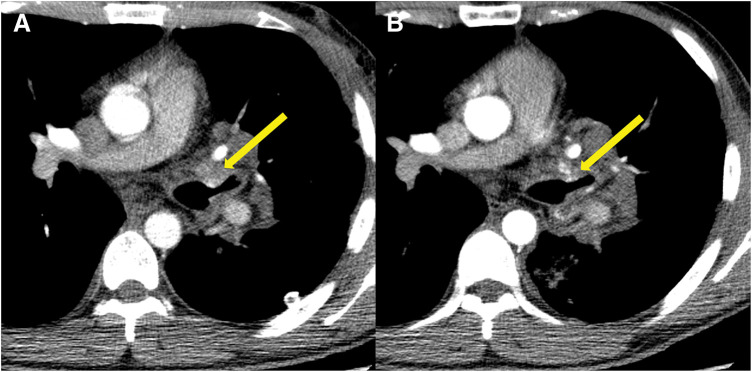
The shrinkage of the AVM on contrast-enhanced CT. CT showing the AVM (yellow arrow) on (A) postoperative day 2 and (B) day 30. AVM: arteriovenous malformation; CT: computed tomography
